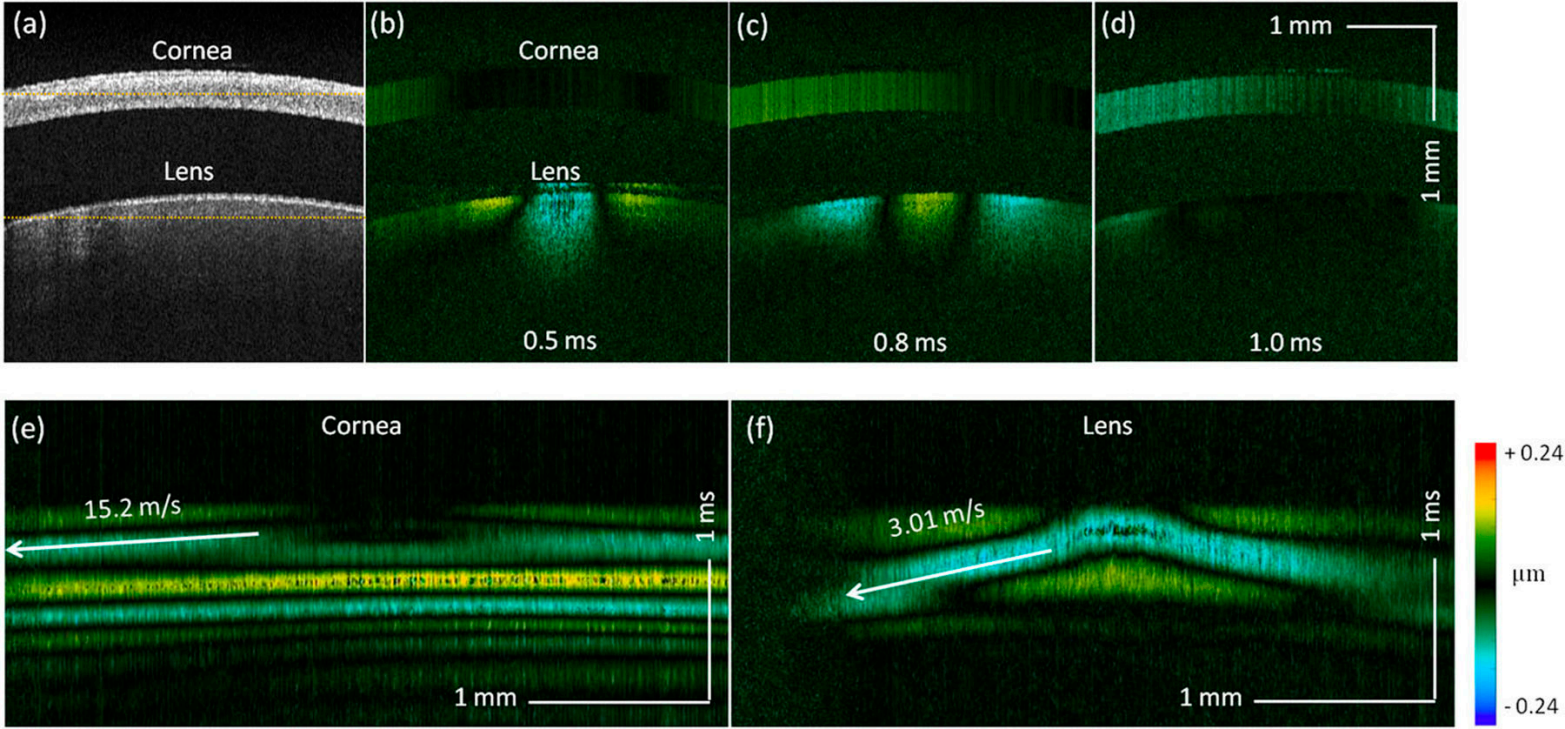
Simultaneous measurements of the lens and cornea in an *in vivo* rabbit model. (a) OCT B-scan images of the lens and the cornea. [(b)–(d)] Doppler OCT B-scans of lens and cornea at 0.1 ms, 0.4 ms, and 0.7 ms after ARF excitation, respectively. [(e) and (f)] Spatiotemporal Doppler OCT of the cornea and lens, respectively, at the depth indicated by yellow dashed lines in (a). Multimedia view: [(b)–(d)]: https://doi.org/10.1063/1.5118258.1
